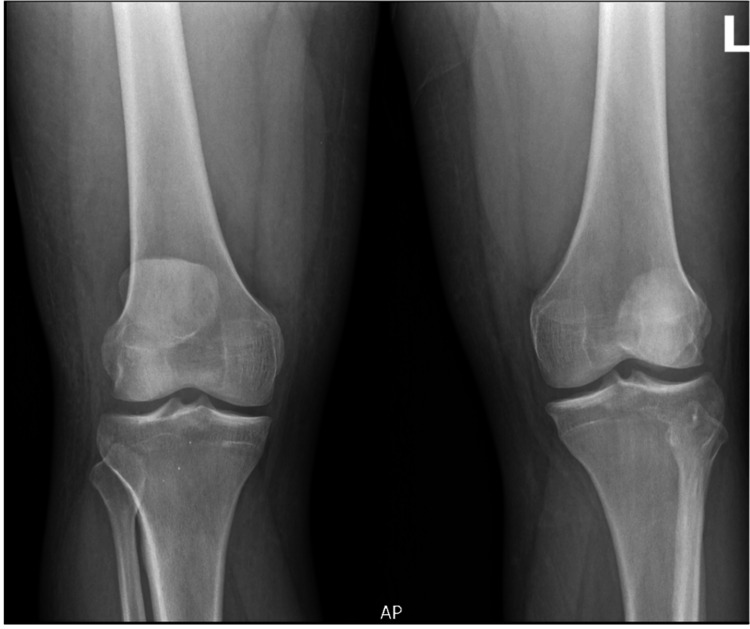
Preoperative AP radiograph of bilateral knees Anteroposterior (AP) radiograph of bilateral knees demonstrating preserved joint spaces with no evidence of a fracture or degenerative changes. Findings are consistent with an extensor mechanism injury.
